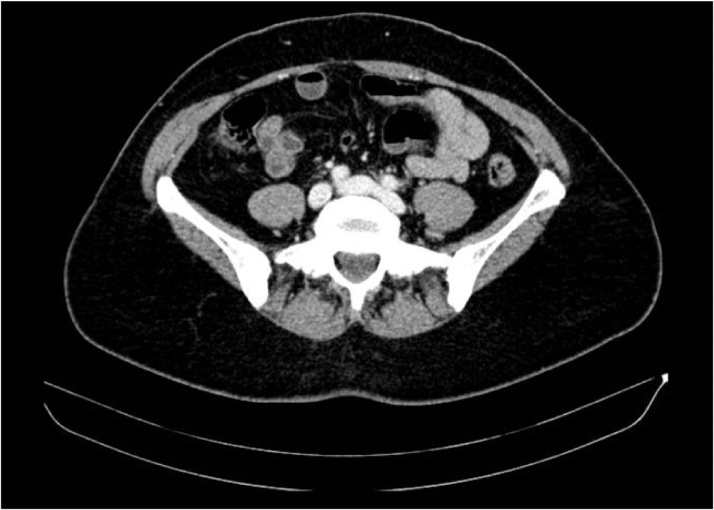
CT scan of the abdomen and pelvis 3 years later, demonstrating no recurrence.
